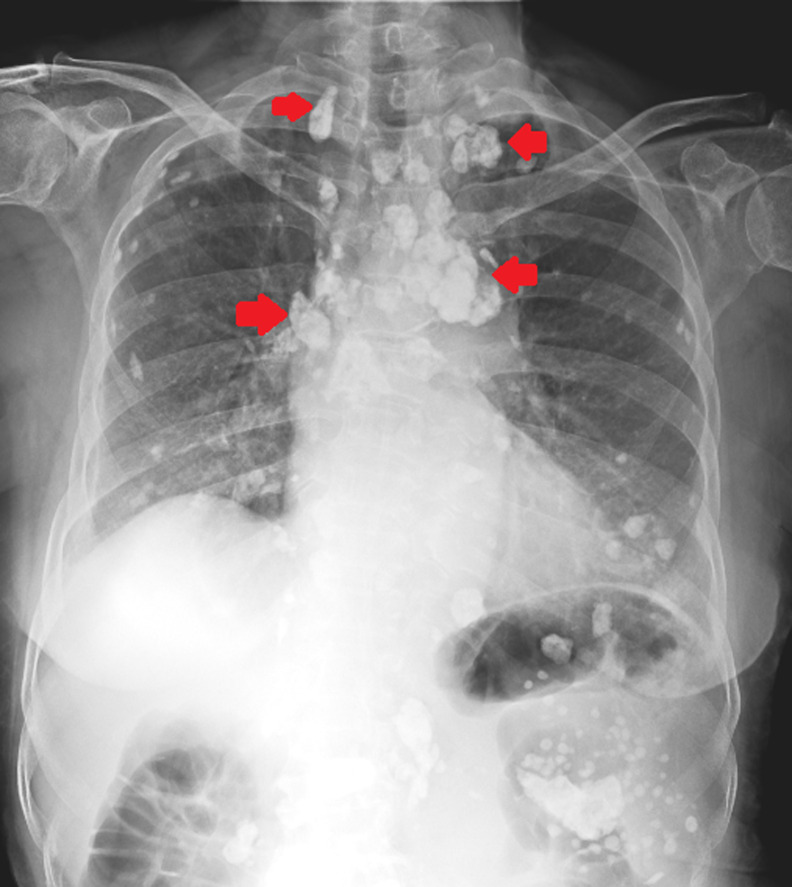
chest X-ray postero-anterior (PA) view showing extensive mediastinal calcifications (red arrows) with diffuse lung calcifications secondary to lymph node tuberculosis
